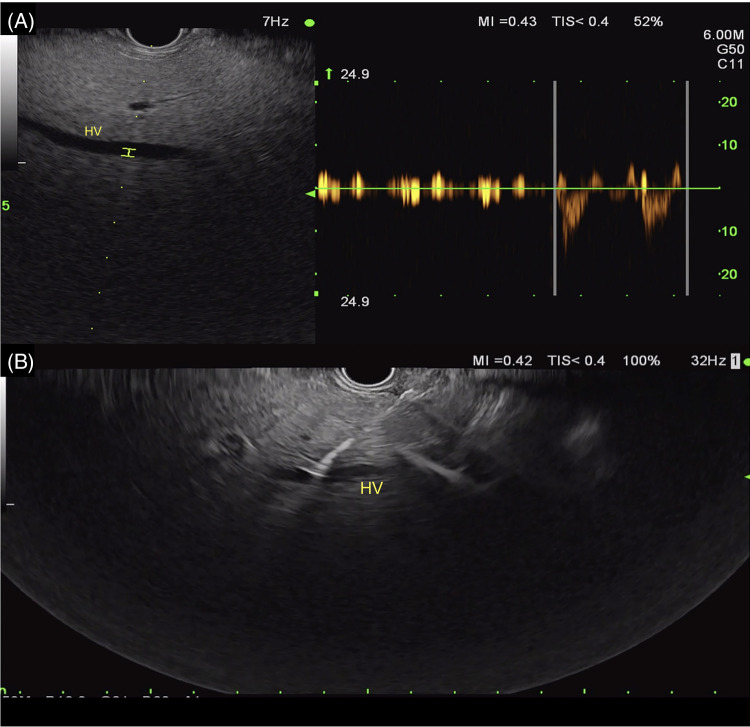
EUS-guided portal pressure measurement of the middle hepatic vein. Abbreviation: EUS, endoscopic ultrasound.
